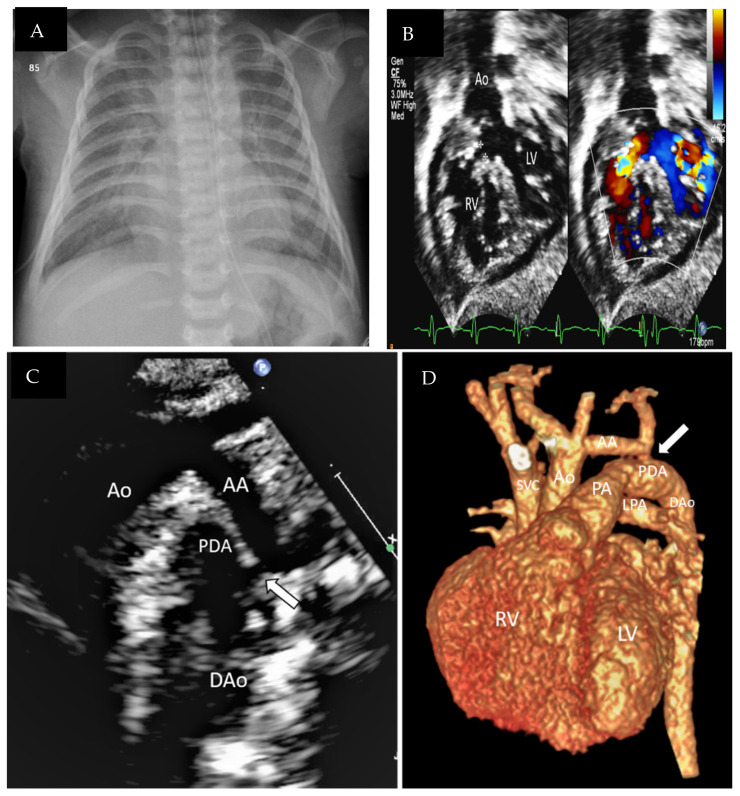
(A) Chest X-ray showing cardiomegaly and congested lungs. (B): Subcostal 2D and color compare echocardiographic view showing the subaortic VSD (* border of VSD). Ao, aorta; LV, left ventricle; RV, right ventricle. (C) Two-dimensional sagittal echocardiographic view of the aortic arch, and 3D reconstructed CT angiographic image (D) showing hypoplasia of the aortic arch with coarctation of the aorta (arrow) and a large PDA supplying the descending aorta. AA, aortic arch; Ao, aorta; Dao, descending aorta; LPA, left pulmonary artery; PA, pulmonary artery; PDA, patent ductus arteriosus; LV, left ventricle; RV, right ventricle; SVC, superior vena cava.
